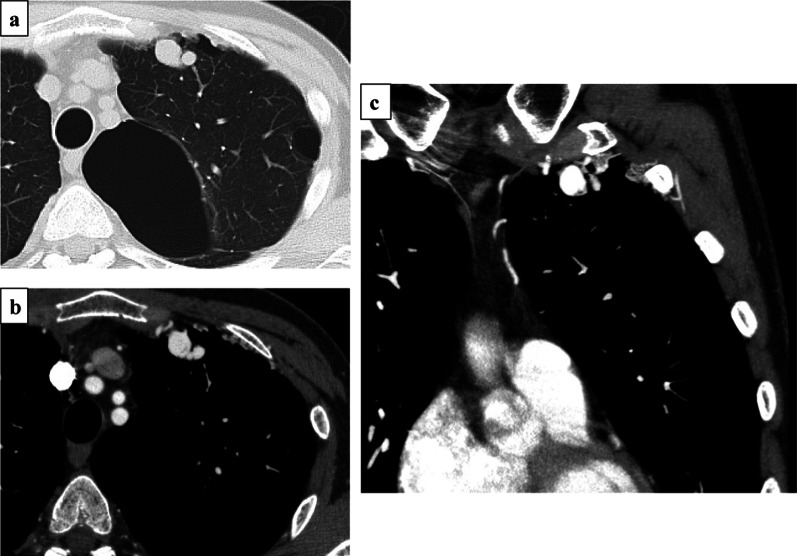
Chest contrast-enhanced computed tomography (CT) scan showed a series of dilated vessels forming a mass-like structure in the anterior segment of left upper lobe. There was a huge bulla at the apex of the lung
